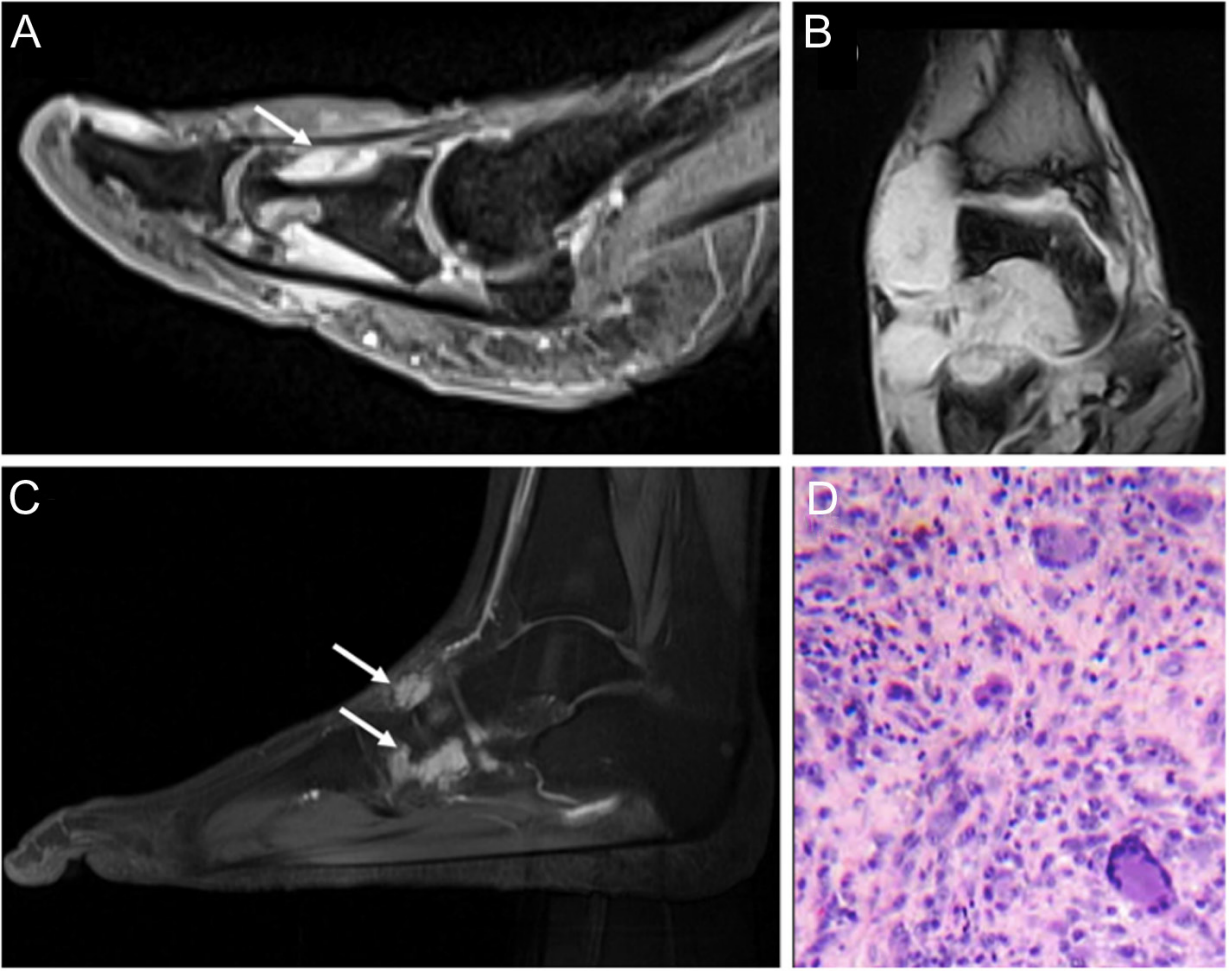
(A) Tenosynovial giant cell tumor (TSGCT) of the right flexor hallucis longus, imaging appearance with MRI in sagittal projection, showing gadolinium-enhancement due to the abundance of capillaries in the collagenous stroma (white arrow); (B) TSGCT of the ankle with aggressive behavior in a 56-year-old female; imaging appearance at MRI with extensive bone and soft tissue involvement (C) Recurrence 12 years after treatment of TSGCT of the foot in 34-year old female patient (white arrows). (D) High power view of a TSGCT, localized type: osteoclast-like giant cells, abundant mononuclear cells, and hemosiderin pigment in the top left.
